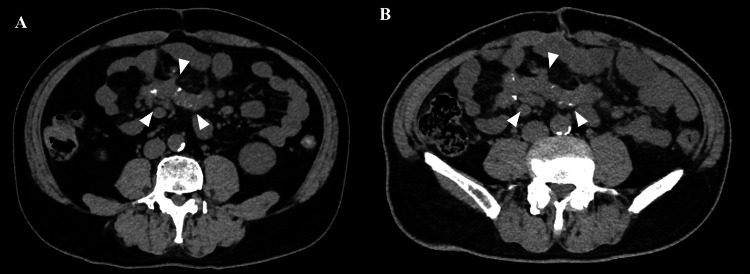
Plain abdominal computed tomography (CT). Plain abdominal CT scans performed at 2.5 years (A: June 2023) and 3.5 years (B: June 2024) after the definitive diagnosis revealed no change in the morphology or size of the branching lobe-shaped lesions (white triangles).
